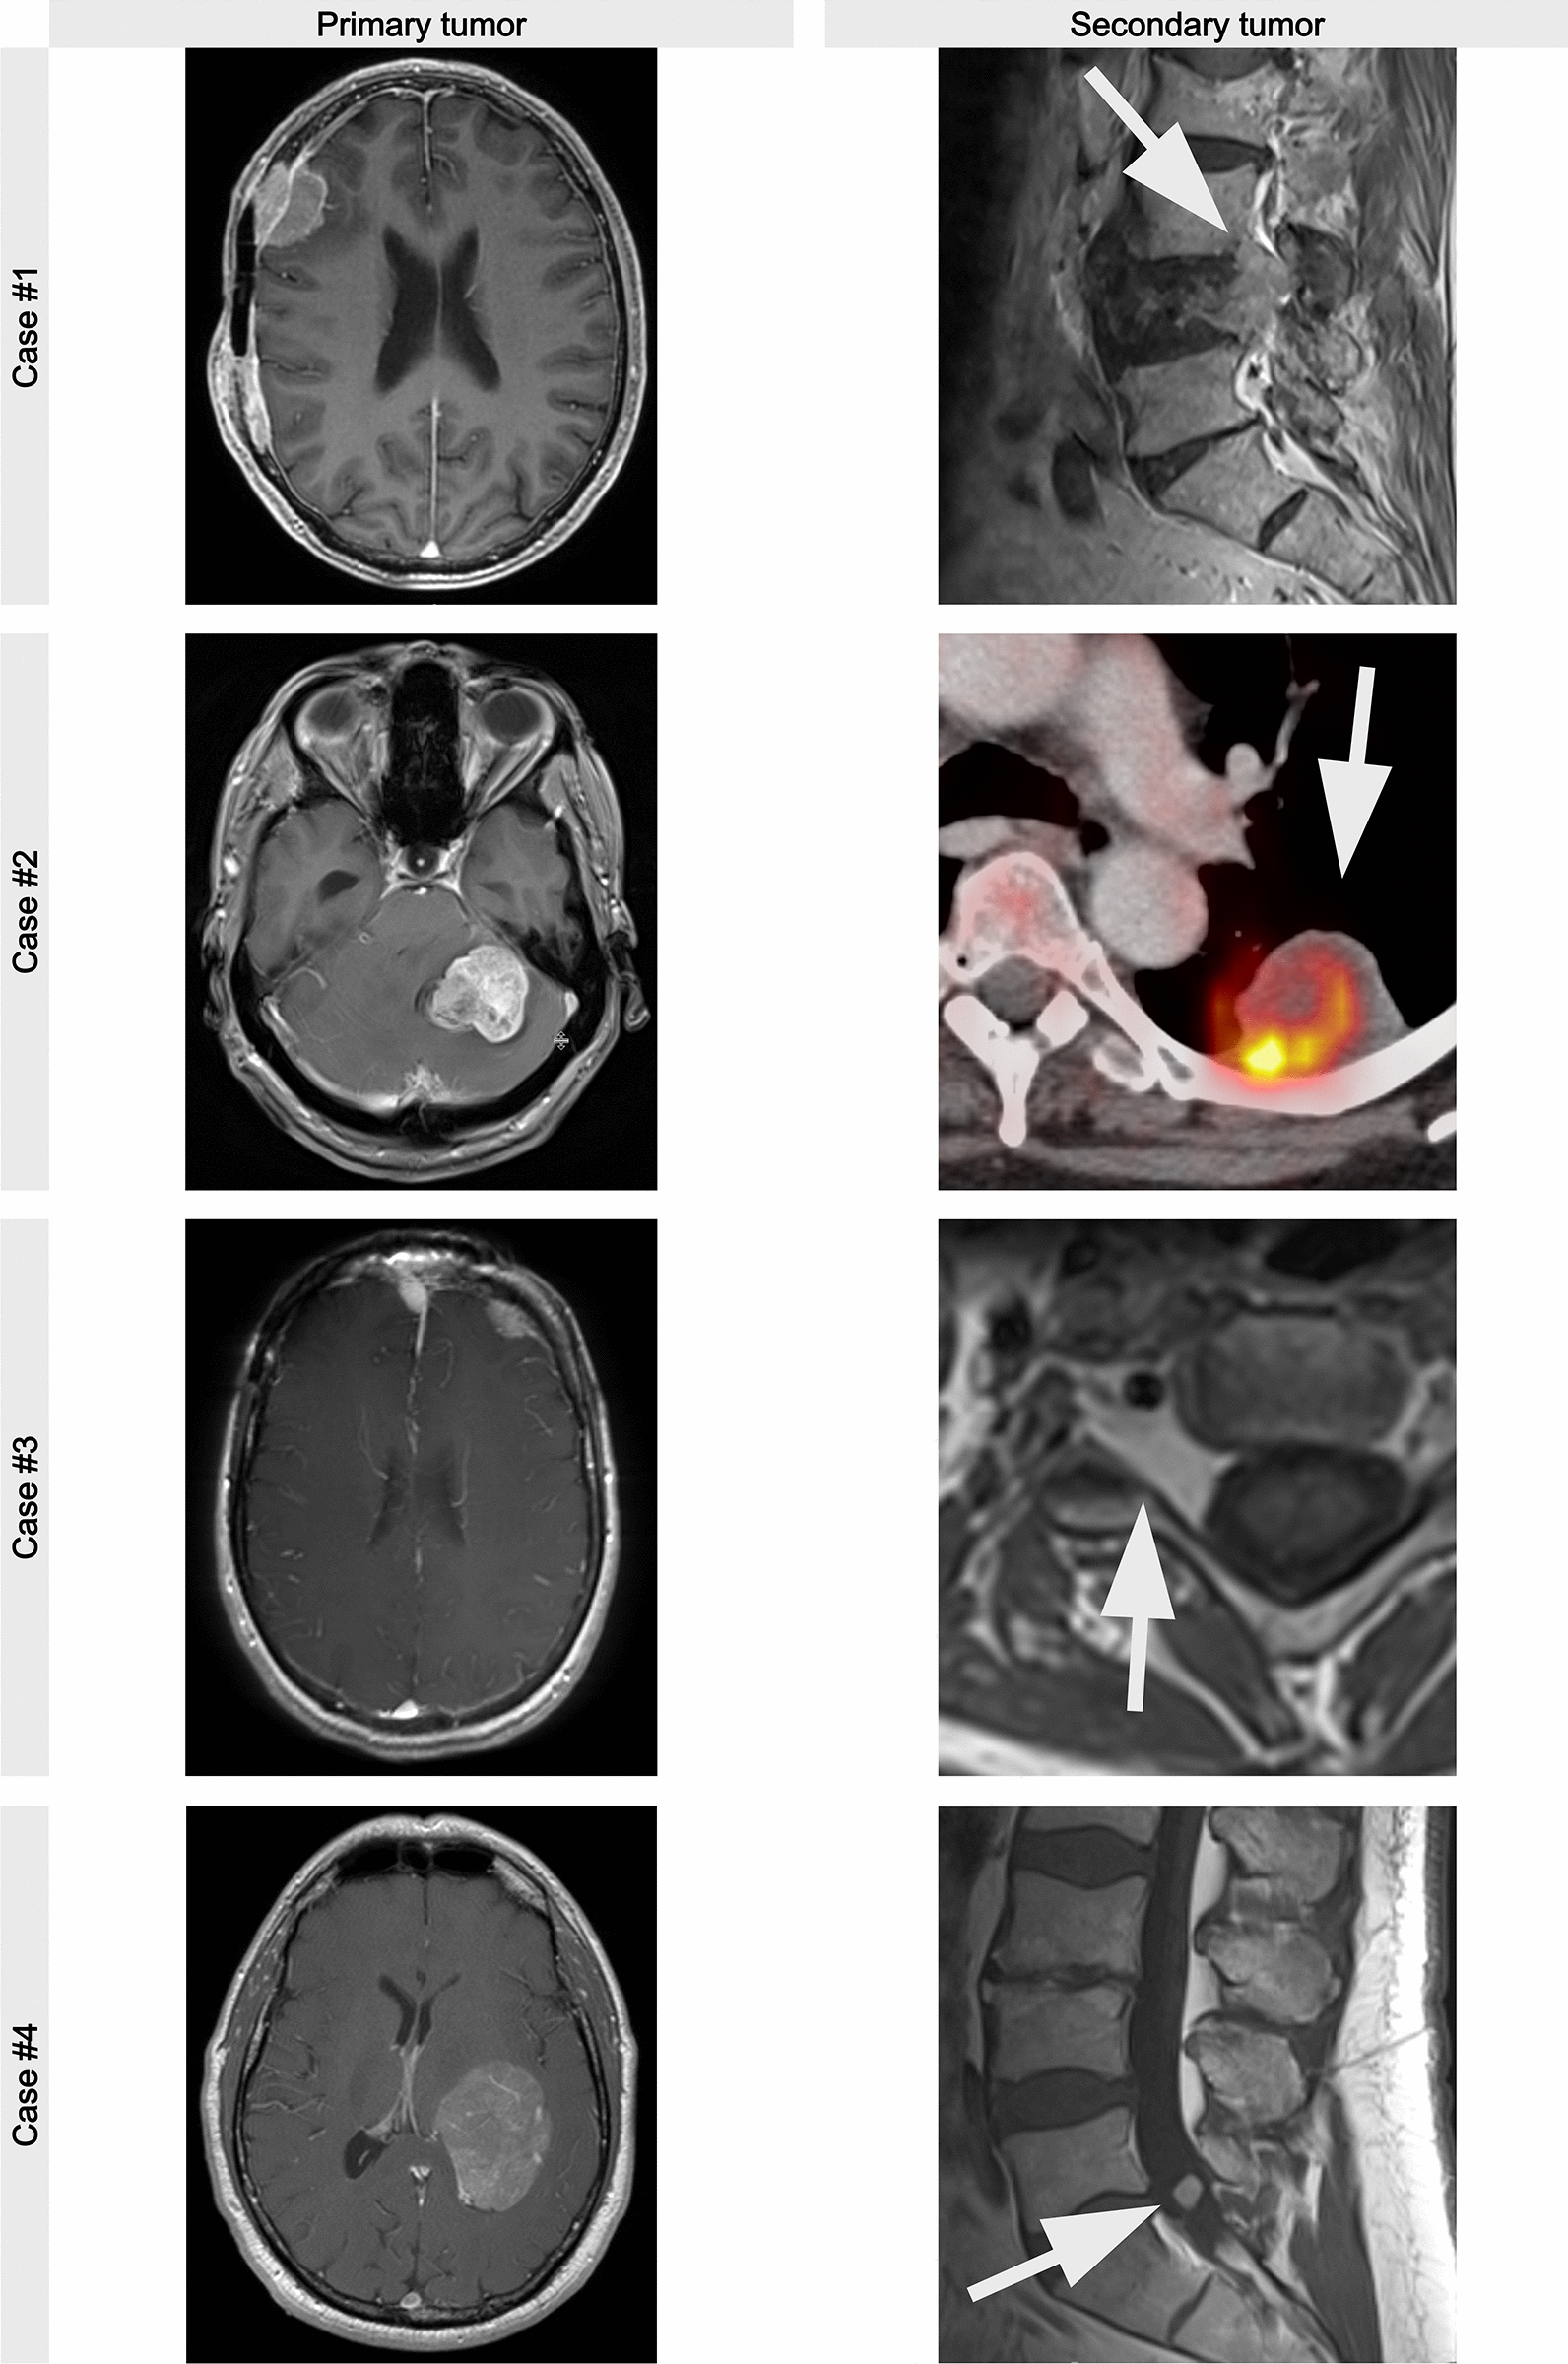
Representative radiographic images of the primary tumor (left column) and respective metastases (right colum)
